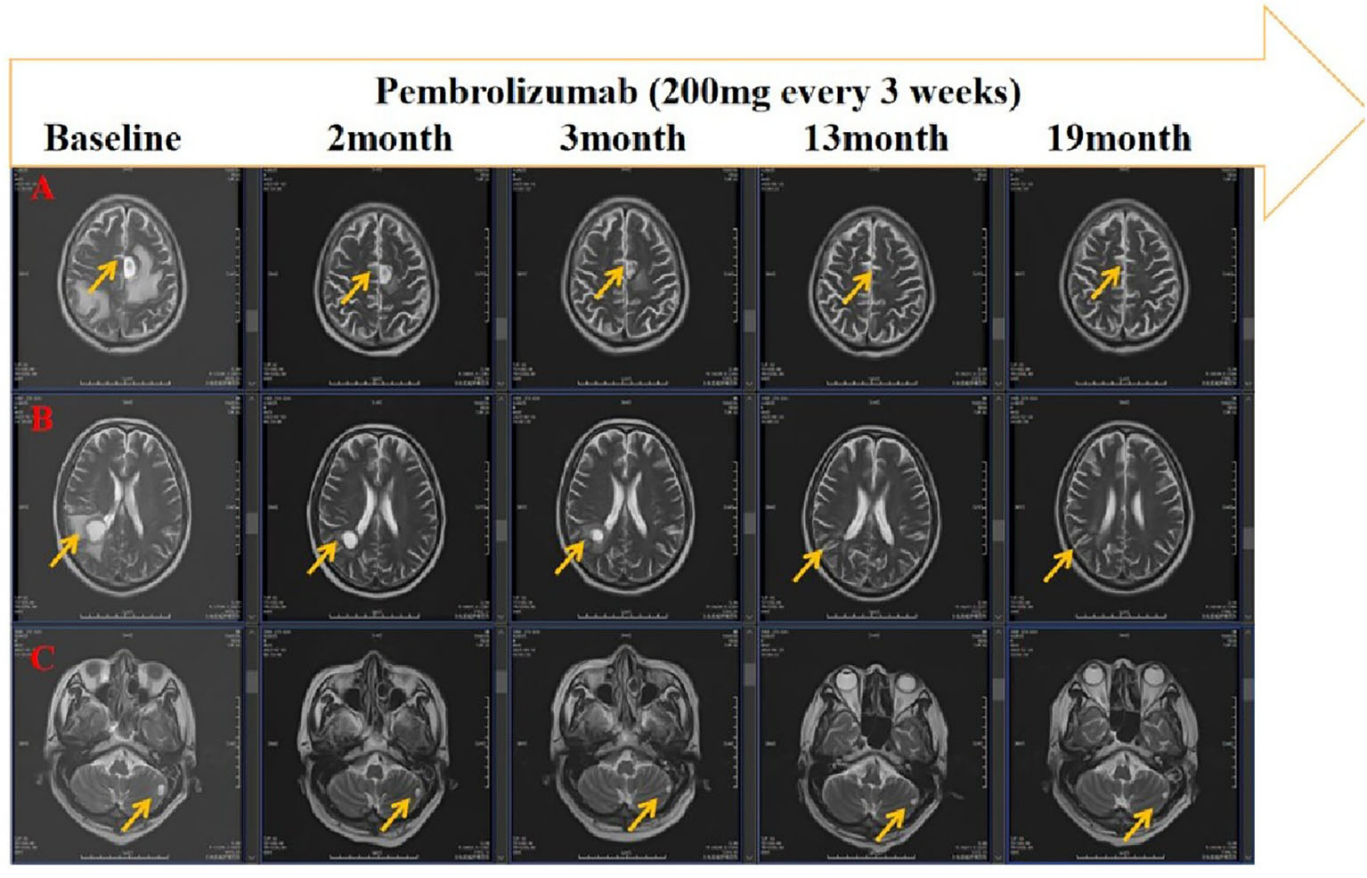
After treatment, head magnetic resonance imaging(MRI) indicated sustained partial response of the left frontal lobe (A). right lateral ventricle posterior horn (B) and left cerebellum (C) lesions.
